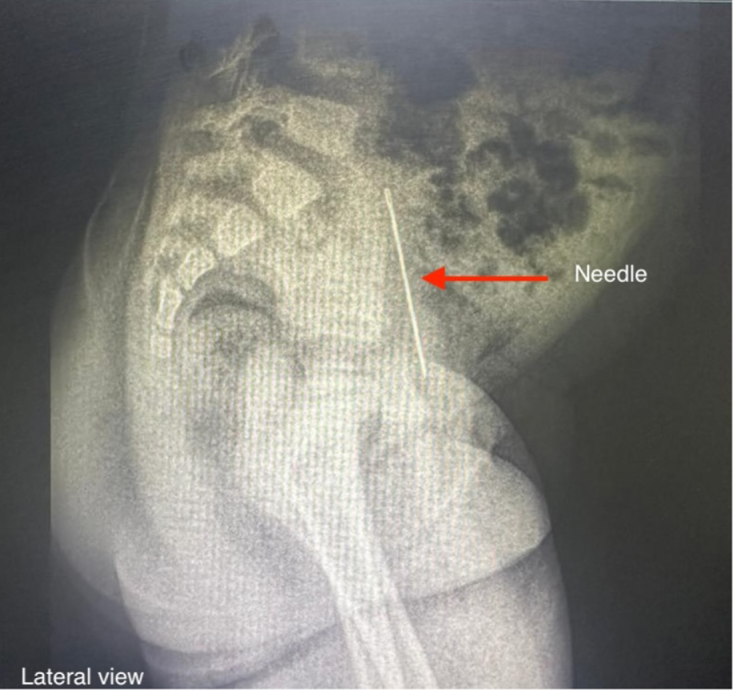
Lateral radiograph demonstrating the intravesical sewing needle (arrow), with clear visualization of its position, orientation, and relationship to adjacent pelvic structures.
